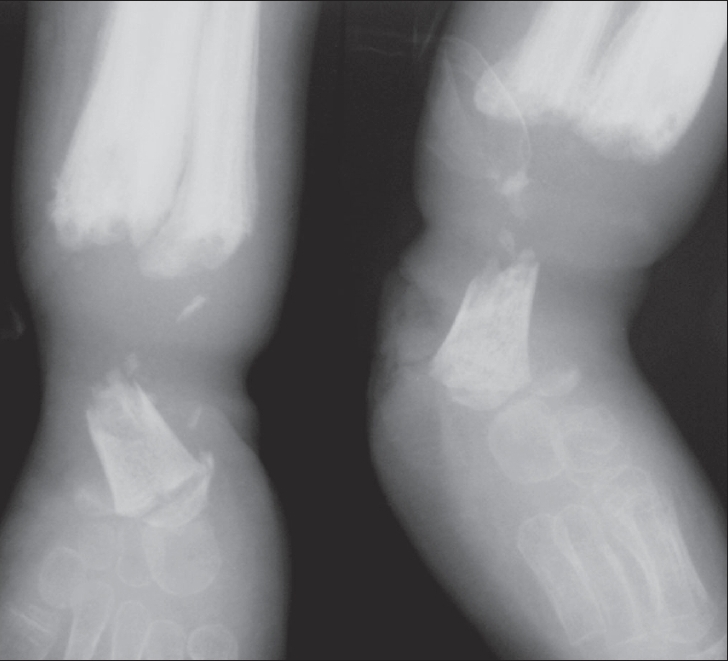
Case 2: Radiograph of the left ankle shows chronic osteomyelitis with periosteal reaction of the first metatarsal bone (arrow) and the lower ends of the tibia and fibula. The latter reveal marked destruction with fracture non-union of tiny sequestra
